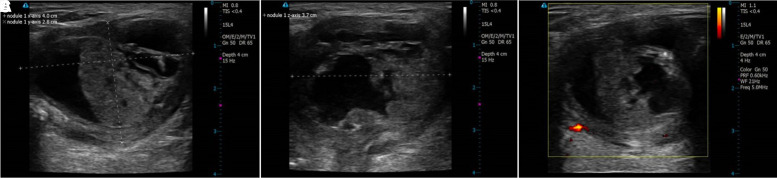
Neck ultrasonography 1 month after ethanol ablation. A and B, transverse and longitudinal views. C, Doppler ultrasound.
